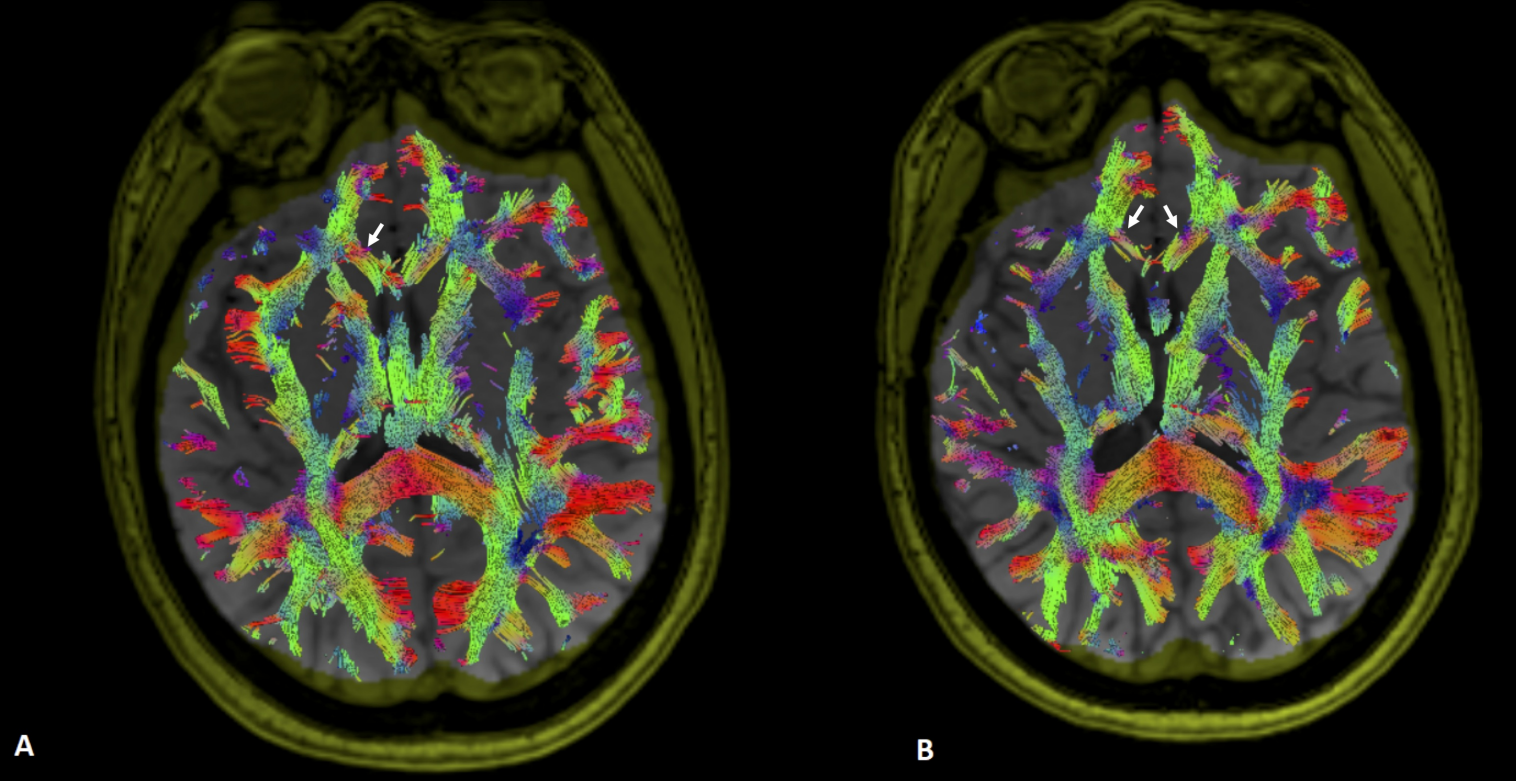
Comparison of pre- and postoperative changes in deep white matter tracts in a patient with right temporal lobe epilepsy. A) Preoperative diffusion tensor fibre tracking of the corpus callosum in a patient with right mesial temporal sclerosis and epilepsy is shown overlaid on T1-weighted axial magnetic resonance imaging (MRI). Preoperatively, the corpus callosum fibres within right minor forceps demonstrate thinning compared to the left (arrow); B) Tracts were analyzed in the same patient after anterior temporal lobe resection. Diffusion tensor fibre tracking exhibits further thinning of the tracts within the minor forceps of the corpus callosum ipsilateral to the resection side (right arrow). Additionally, the left forceps minor demonstrates increased thinning of tracts not seen preoperatively.
